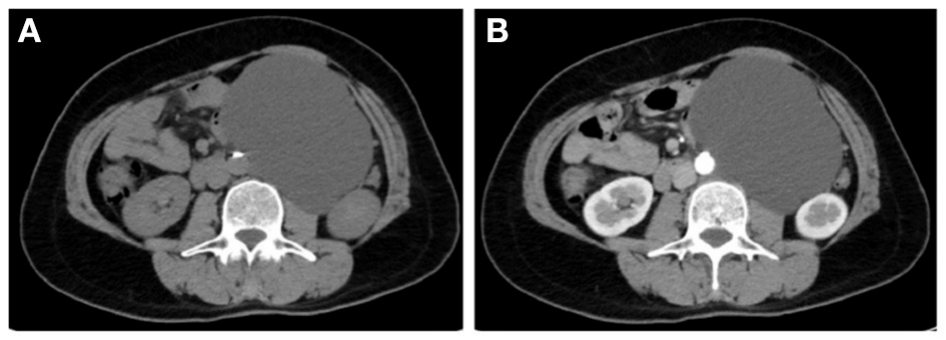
CT showed a large thin-walled cystic mass of low density (A) with no enhancement (B).
